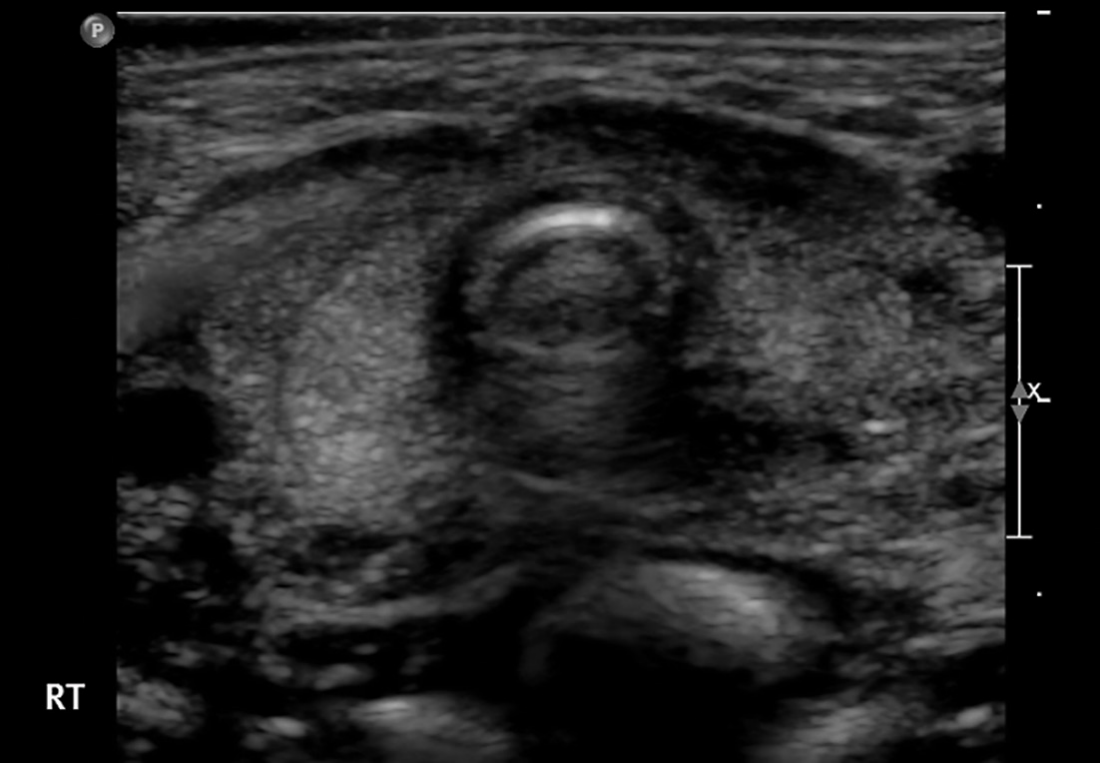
Ultrasound image showing perithyroid oedema due to allo-immune thyroiditis in an infant born to a mother with very elevated TSH-receptor antibodies (>405 u l^−1^). Neither subjective evaluation nor objective measurement was possible in this patient.
